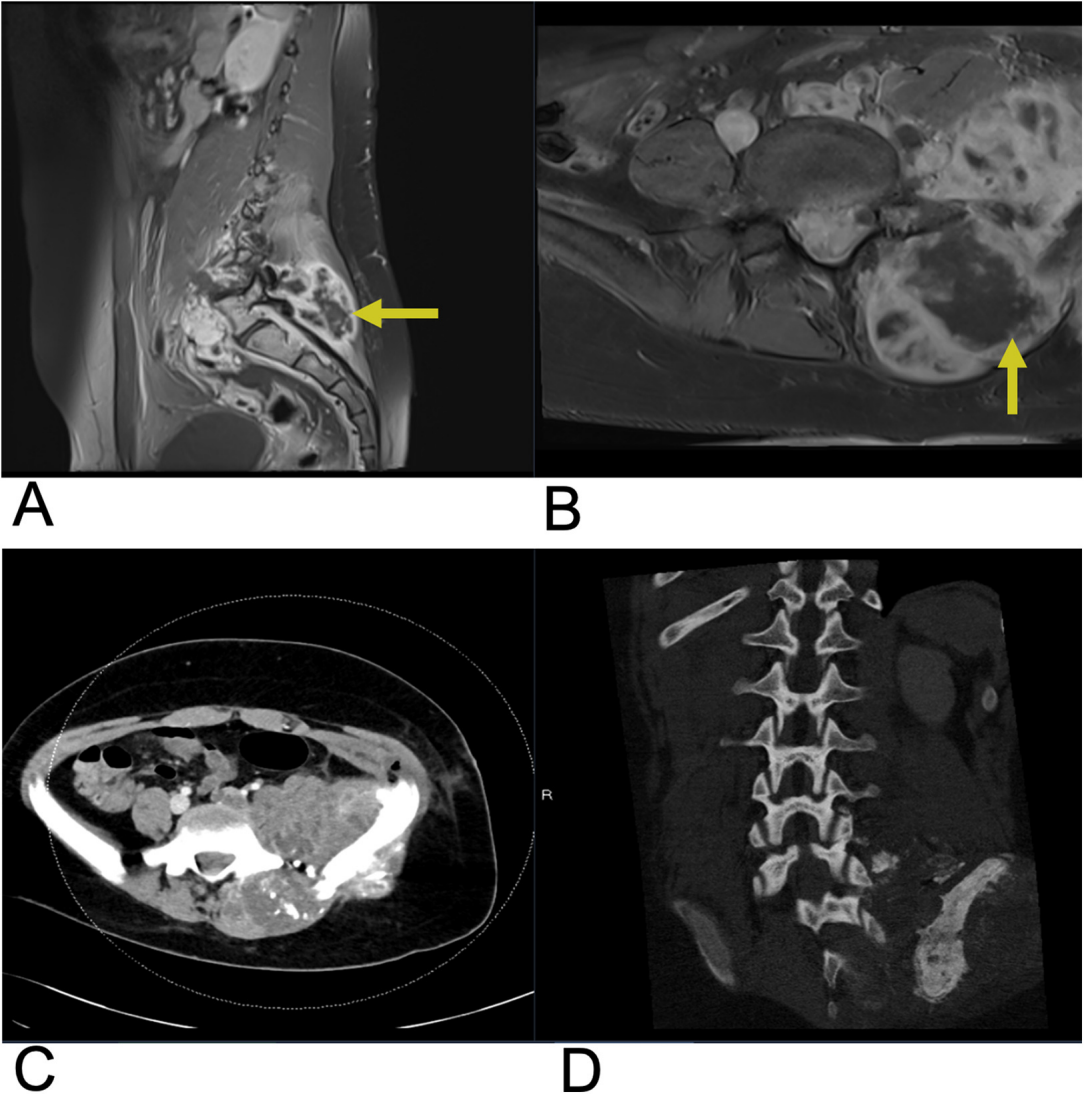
Osteosarcoma. Sagittal T1 + C (A), Axial T1 + C (B) MRI as well as axial CT (C) and sagittal CT (D) of the lumbar spine demonstrating a large, destructive mass in the sacral region (yellow arrows) indicating osteosarcoma with adjacent soft tissue involvement.
